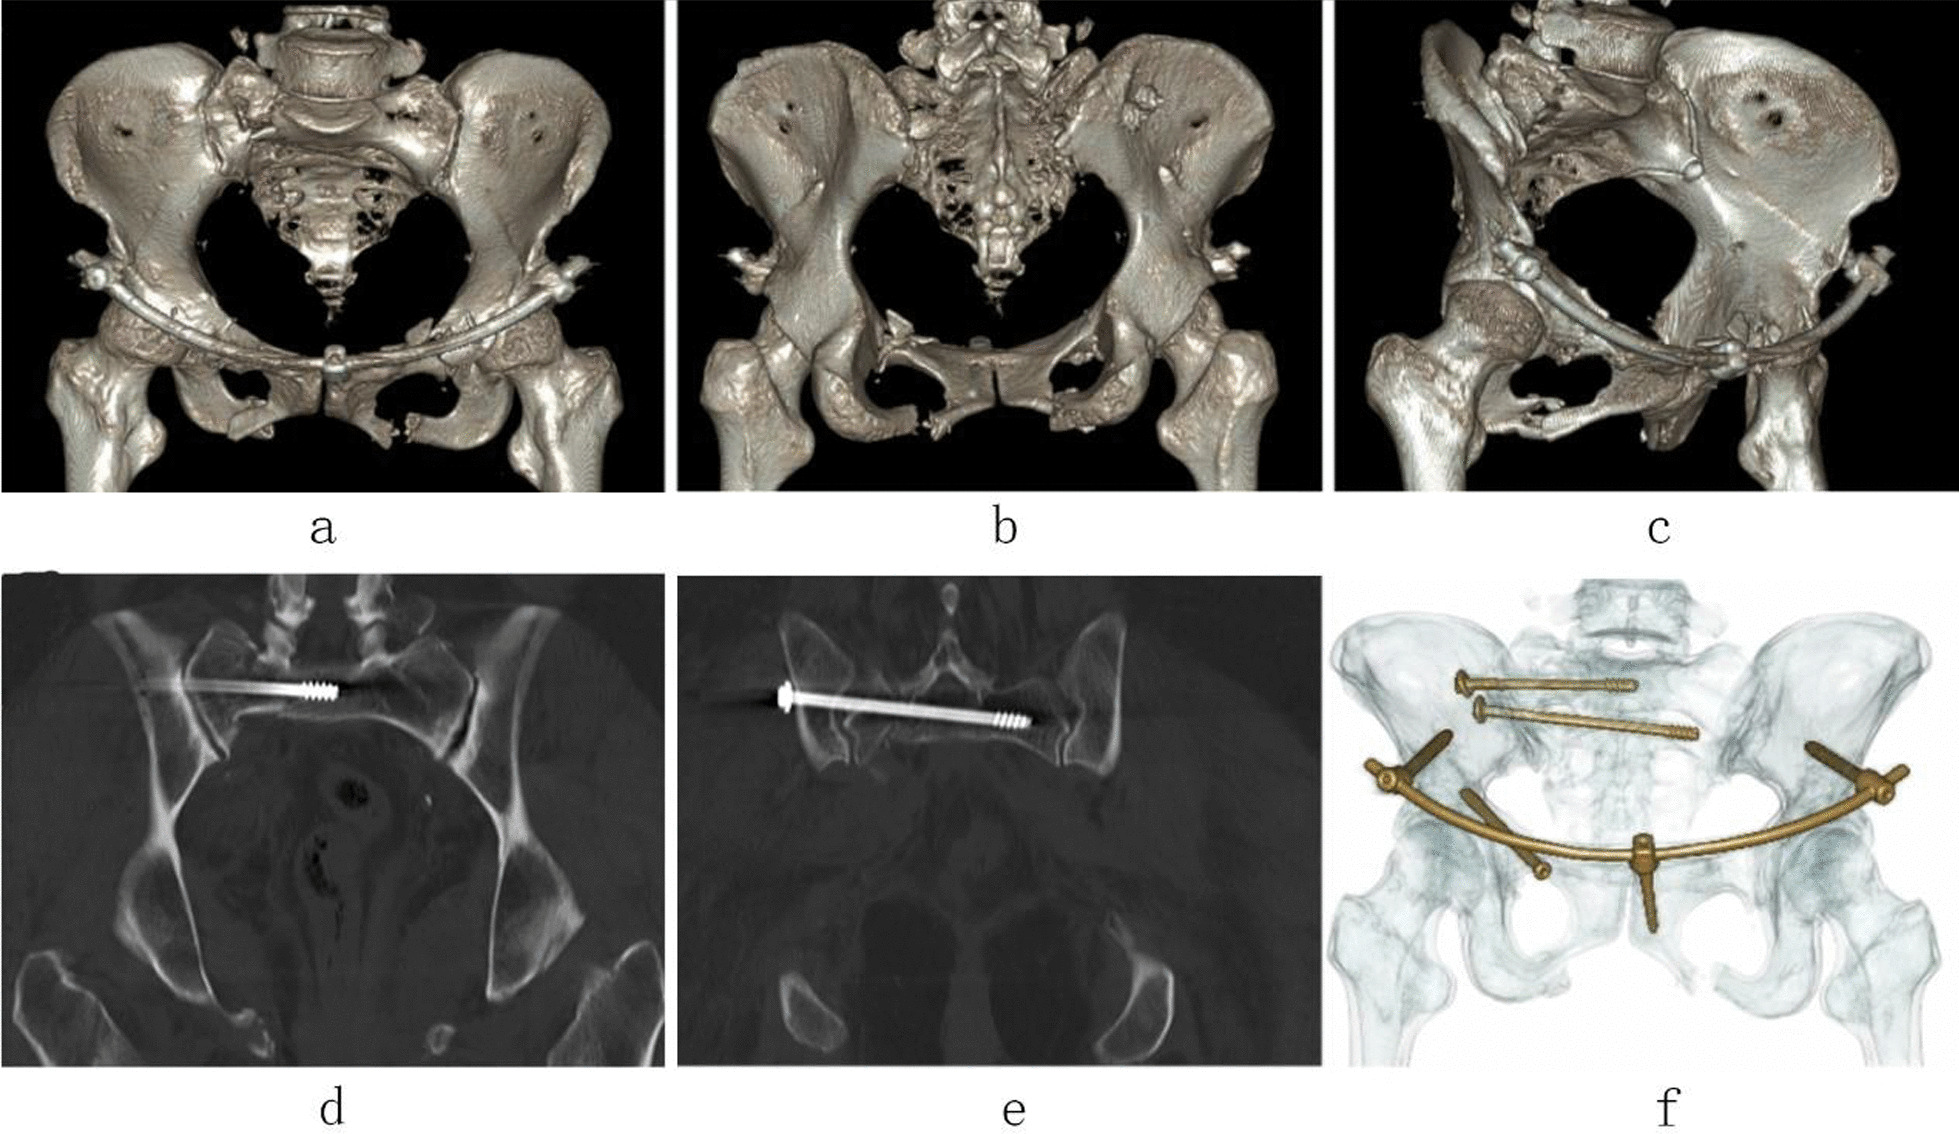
Postoperative CT images of case 2. (a–c, f CT 3D reconstruction images, d, e CT sagittal image.)
